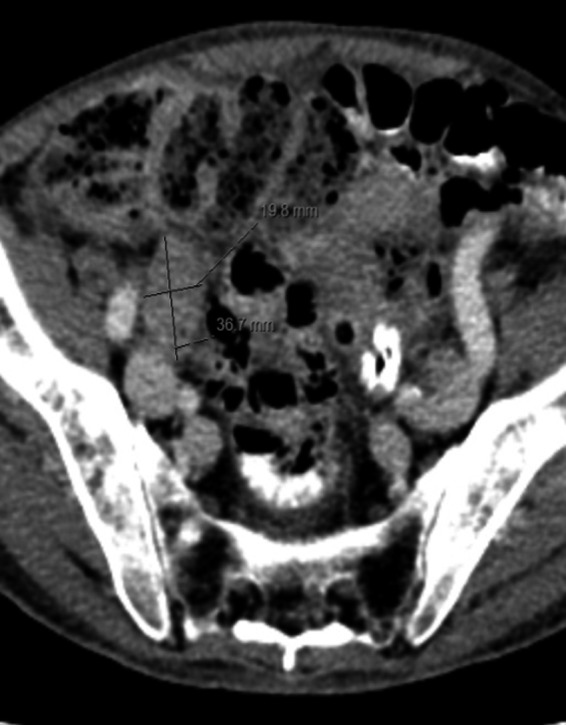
Axial postcontrast CT shows enlarged right iliac lymph node.
